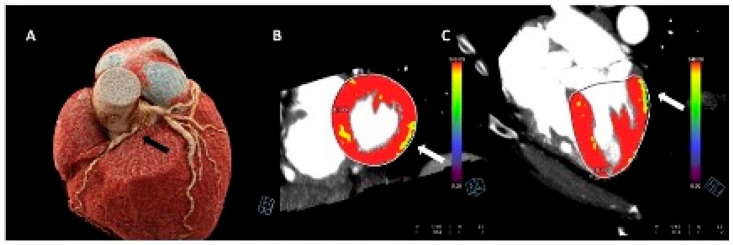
Stress-computed tomography perfusion imaging of a 15-year-old boy with an anomalous origin of the right coronary artery from the left sinus of valsalva. The black arrow indicates the coronary artery abnormality (Panel (A)). The images with arrows (B,C) indicate stress-induced perfusion defects of the infero-lateral wall of the left ventricle.
